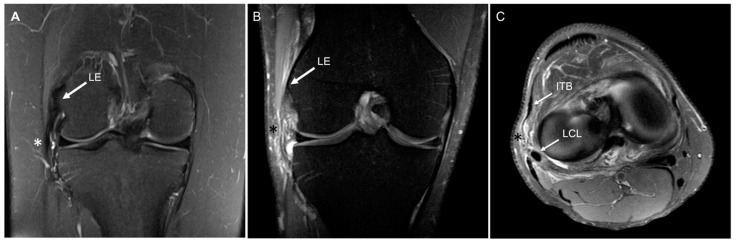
T2 fat suppressed magnetic resonance imaging scans from a right knee with (A) no sign of an anterolateral complex injury on the coronal view (white asterisk). In (B,C), a grade 2 anterolateral complex injury with partial fiber disruption and edema is visible as indicated with the black asterisk. LE, lateral epicondyle; LCL, lateral collateral ligament; ITB, iliotibial band.
